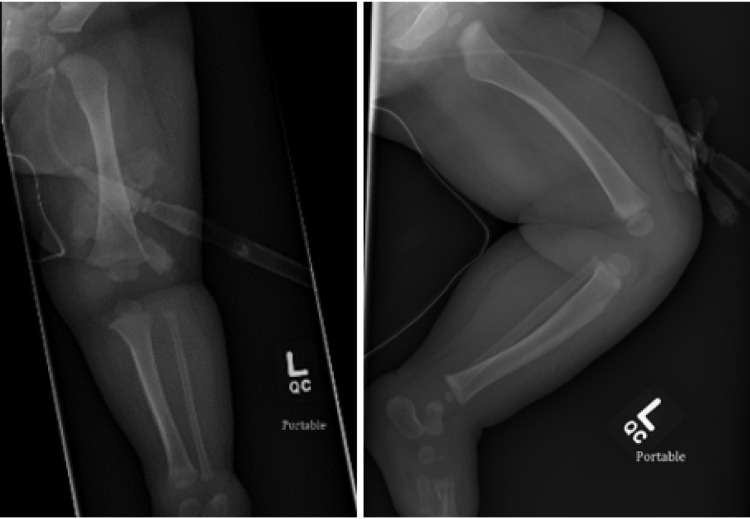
Radiography of the left leg AP (Pane A) and lateral (Pane B) of the left leg, before resection of the fibula, demonstrating extensive soft tissue edema.
